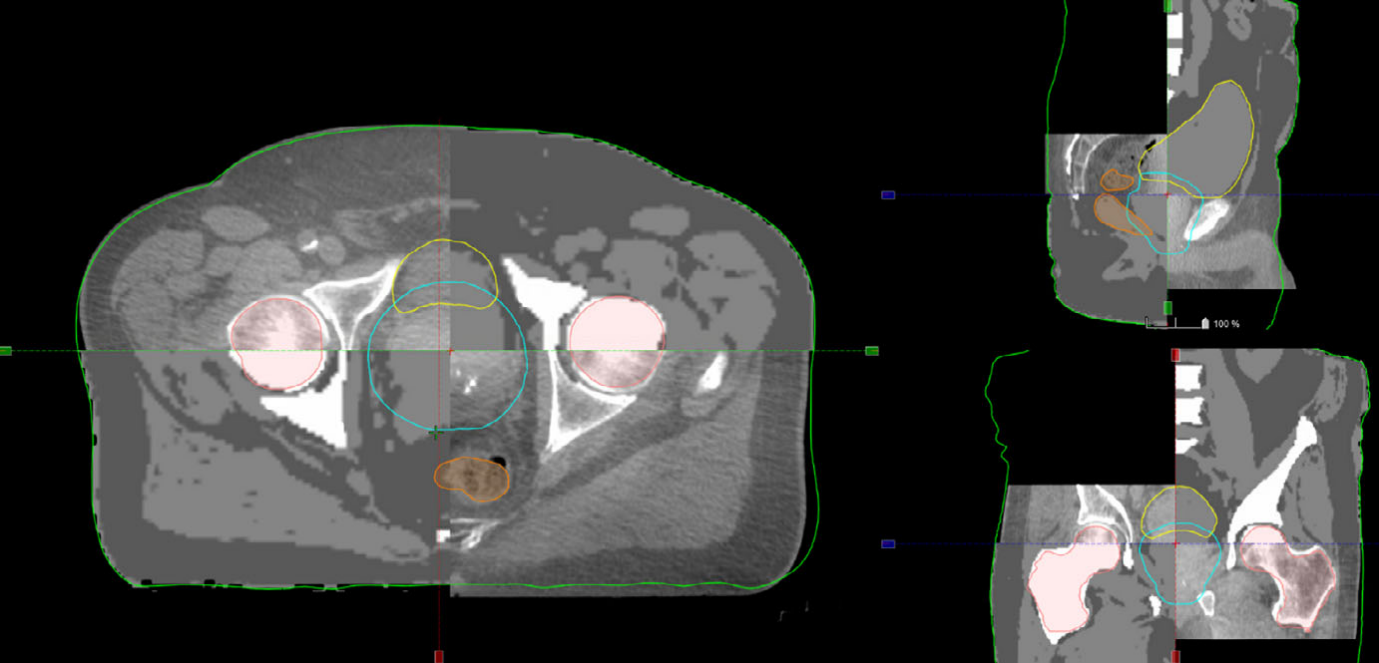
sCT / CBCT comparison. The following structures delineated on the MRI are displayed: outer contour, femoral heads, bladder, rectum and prostate PTV.
